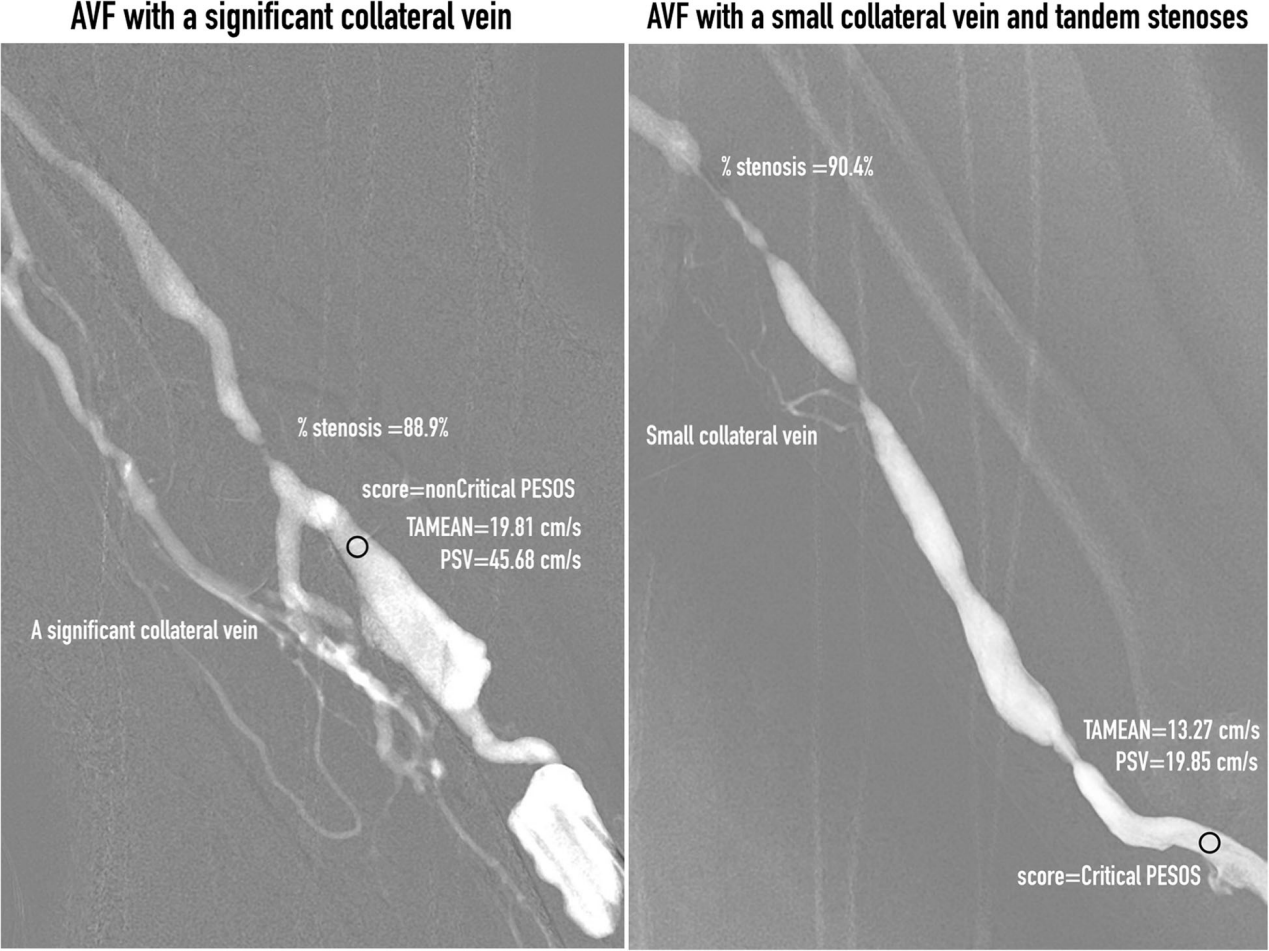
Roadmap angiograms of an AVF with a significant collateral vein (left) and a small collateral vein (right). The back circle on the AVF indicate the score recorded site, where the Doppler flow was measured
